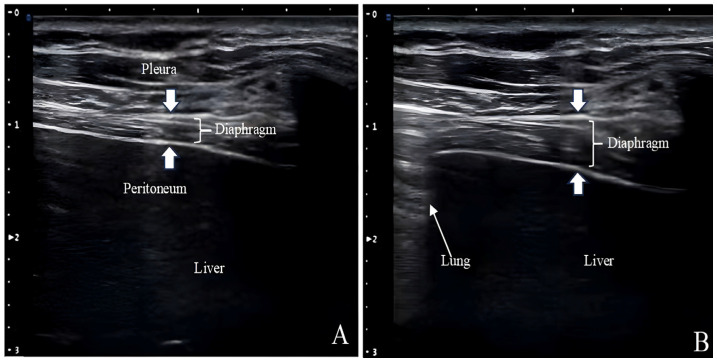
Measurement of diaphragmatic thickness through B-Mode ultrasonography. The distance between the pleura and peritoneum represents diaphragmatic thickness. The images show a change in normal diaphragm thickness between end-exhalation (A) and end-inspiration (B). The lung is visualized at end-inspiration.
